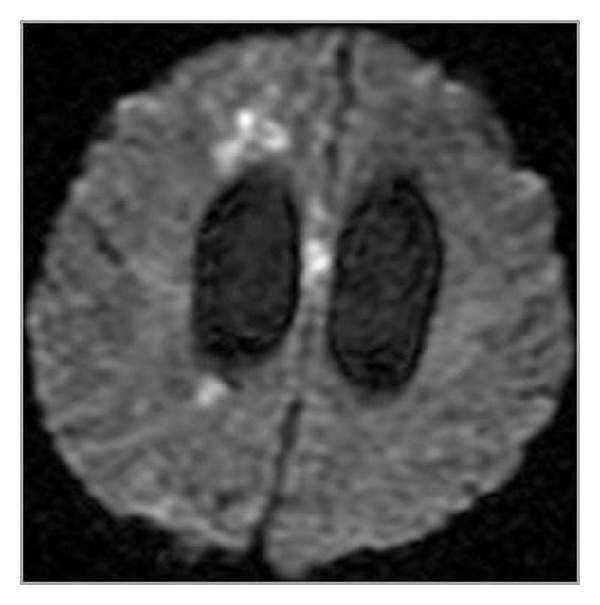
DAI—acute phase visualized on DWI.
